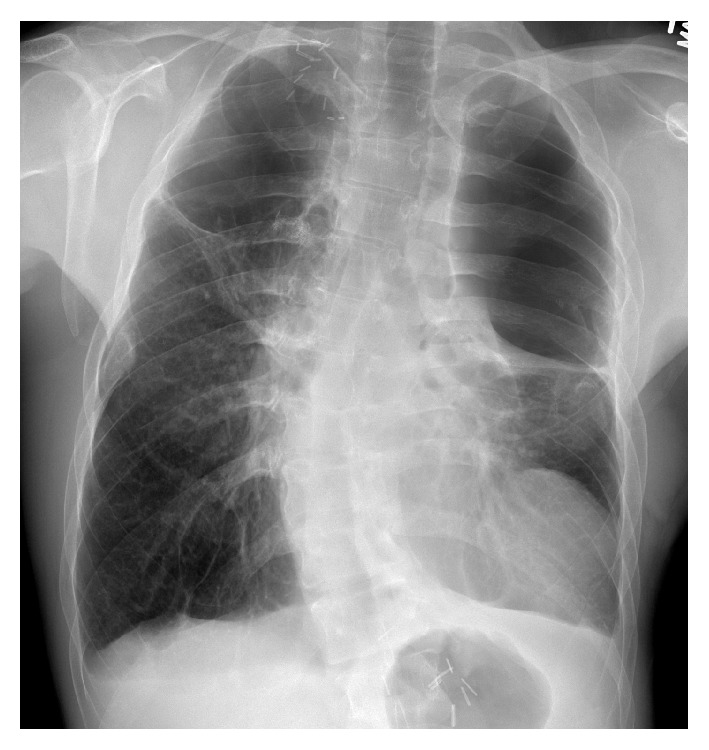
PA chest X-ray taken in July 2013 shows mass lesion at the level of left cardiophrenic recess and biapical large bullae.
